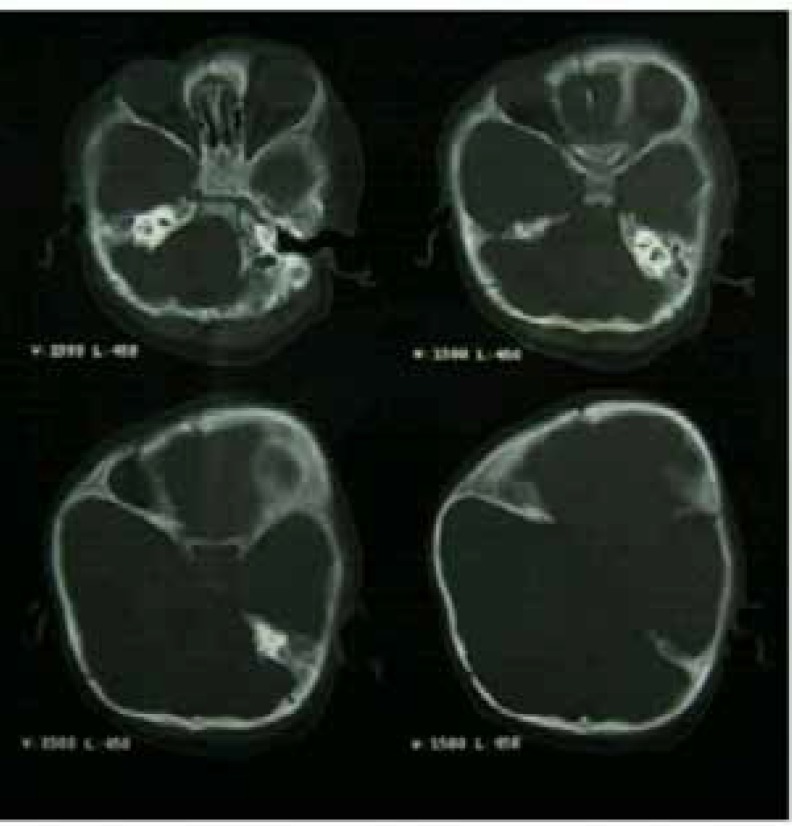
CT scan of cranium and orbit
